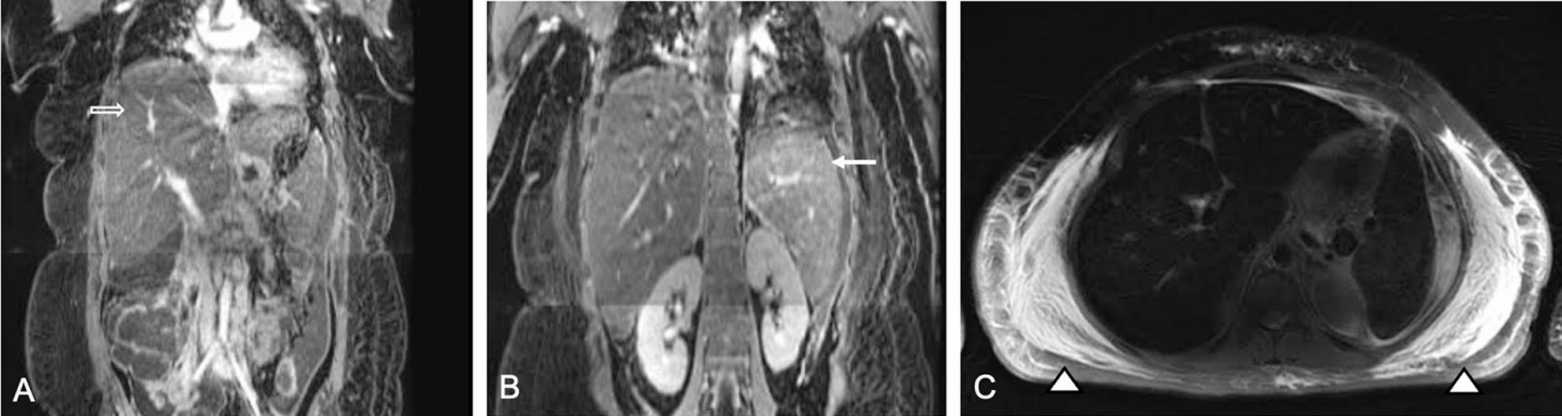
Contrast-enhanced MRI scans of a 18-year-old woman with relapsed B-cell acute lymphoblastic leukemia, who developed hemophagocytic lymphohistiocytosis at day 42 after CAR T-cell therapy. A) Coronal contrast-enhanced T1-weighted MRI shows marked edema and swelling of the liver (open arrow, 23.8 cm in craniocaudal dimension). B) Coronal contrast-enhanced T1-weighted MRI shows marked enlargement of spleen (arrow, 16.2 cm in craniocaudal dimension). C) Axial T2-weighted MRI through the level of the liver and spleen shows marked subcutaneous soft tissue edema (arrow heads) and diffuse low T2-signal of the liver and spleen due to severe iron overload
